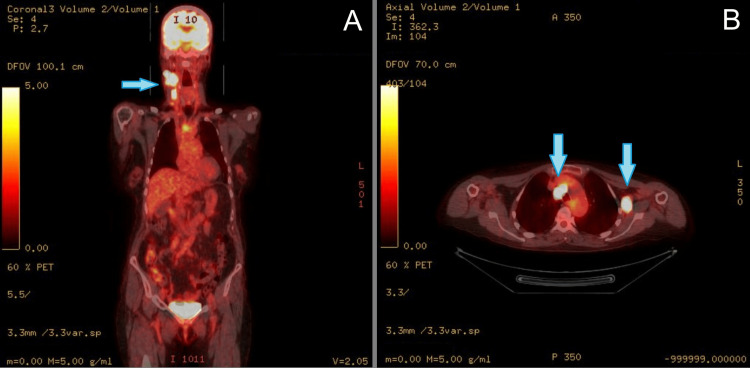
Whole-body FDG PET/CT demonstrating abnormal radiotracer uptake in the right neck, mediastinum, and left shoulder region. Representative FDG PET/CT images at the time of diagnosis demonstrate increased tracer uptake in the enlarged right cervical lymph nodes (Panel A, coronal section), prominent mediastinal lymph nodes (Panel B, transverse section), and enlarged left axillary lymph nodes (Panel B, transverse section), compatible with B-cell lymphoma. Abnormalities are denoted with blue arrows above. The image has been fully de-identified in accordance with the institution's review board (IRB) policy, which does not require patient consent for the use of de-identified clinical data in publications. FDG PET/CT, fluorodeoxyglucose positron emission tomography/computed tomography
